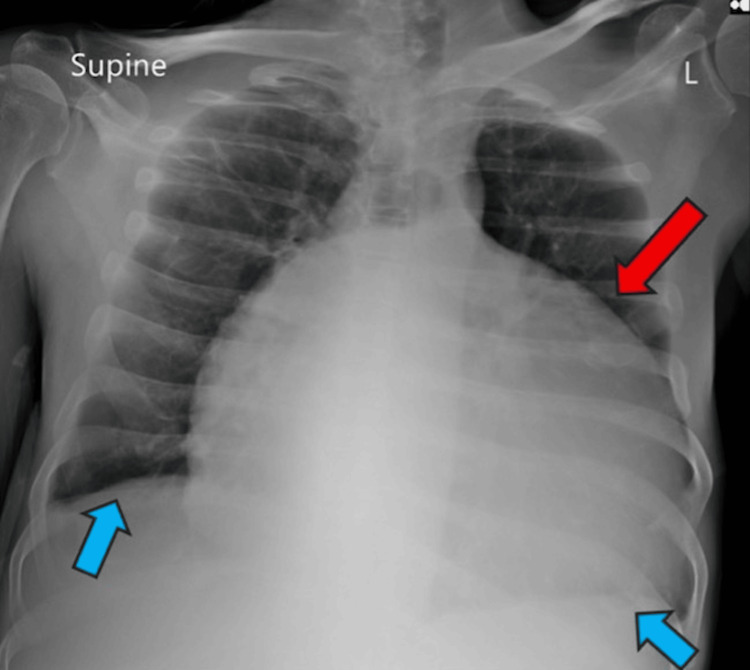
Chest radiograph showing cardiomegaly and pulmonary vascular congestion Portable anteroposterior chest radiograph obtained in the supine position, showing marked enlargement of the cardiac silhouette (red arrow), bilateral pulmonary vascular/interstitial congestion, and flattening of the diaphragms consistent with chronic hyperinflation (blue arrows). No focal air-space consolidation or pleural effusion is identified.
